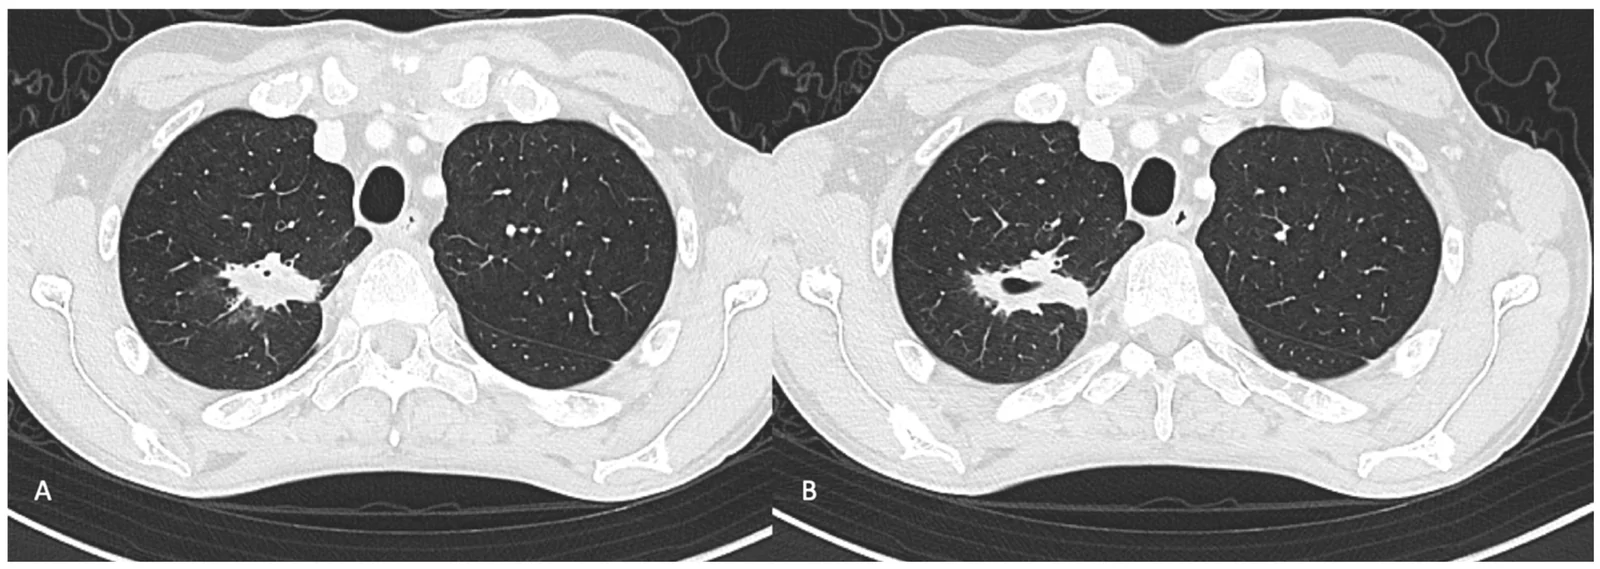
HRCT, axial plane. In this case, the patient had a strong clinical suspicion of bartonellosis and was therefore examined with HRCT rather than CECT. In the first CT scan (A) it appears as a large mass in the right upper lobe. At the one-month follow-up after specific therapy (B), unfortunately, the lesion had cavitated, but it did not evolve into a necrotizing form, so intralesional cavitation was detected but no findings indicative of necrosis—as multiple internal air–fluid levels surrounded by areas of parenchymal consolidation. Without clinical and laboratory data, HRCT findings may be very similar to TB or MAC infection, making radiological diagnosis very challenging.
